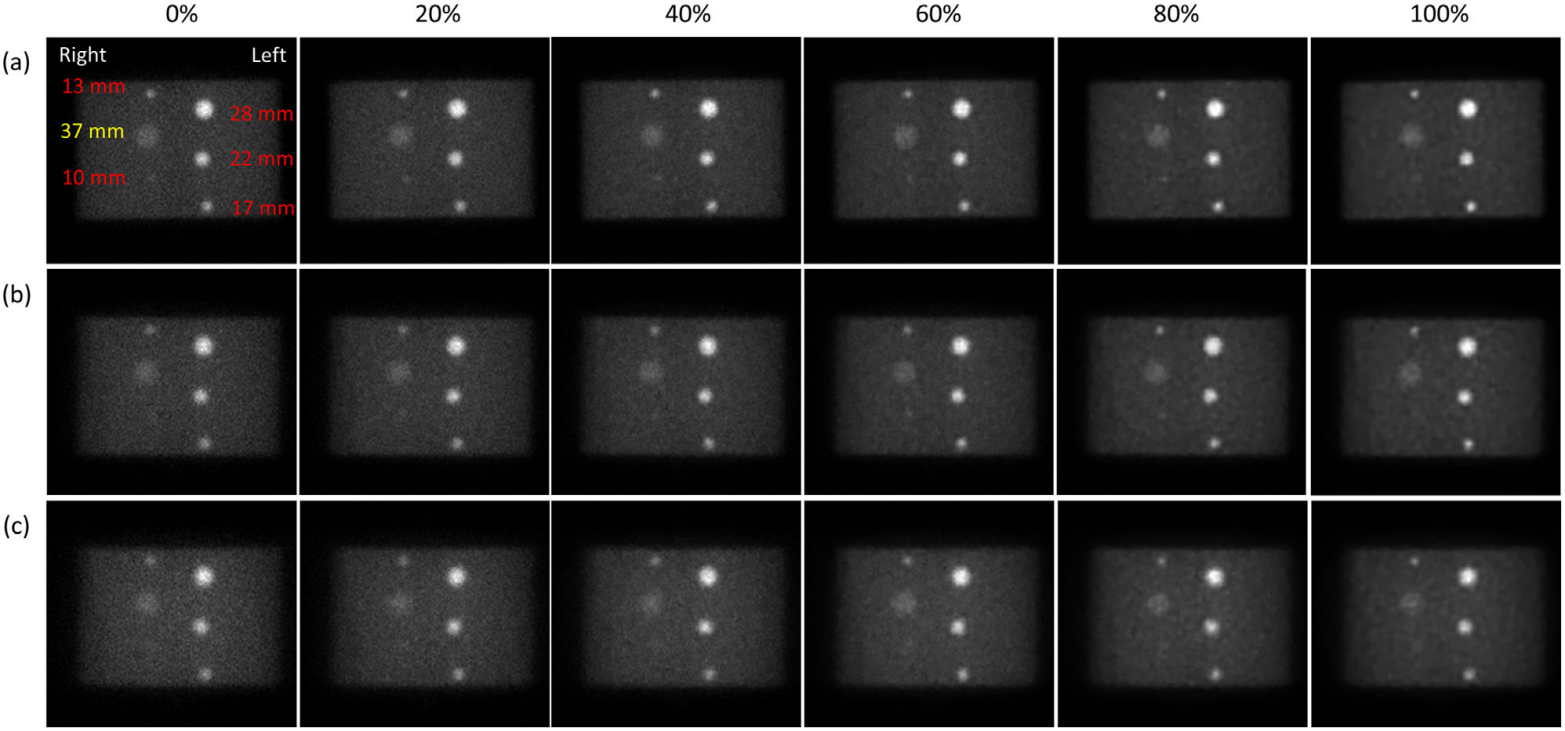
Anterior view of Tc‐99m planar scintigraphy (window level = 100/window width = 200) acquired with a blend ratio of 0%, 20%, 40%, 60%, 80%, and 100% (from left to right) for (a) NEMA_small_, (b) NEMA_medium_, and (C) NEMA_large_
